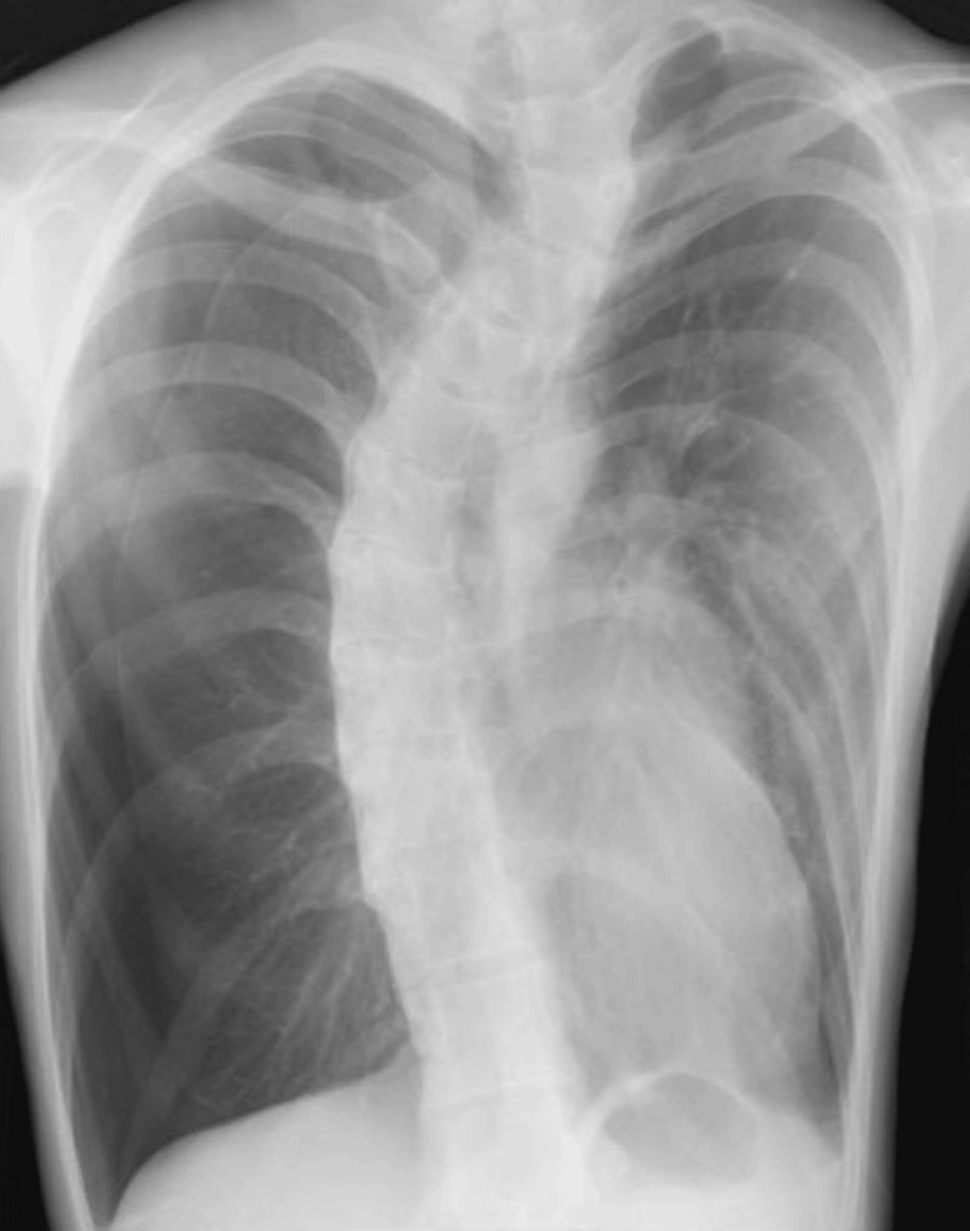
Preoperative chest radiograph. Right pneumothorax was developed. Scoliosis was significant.
